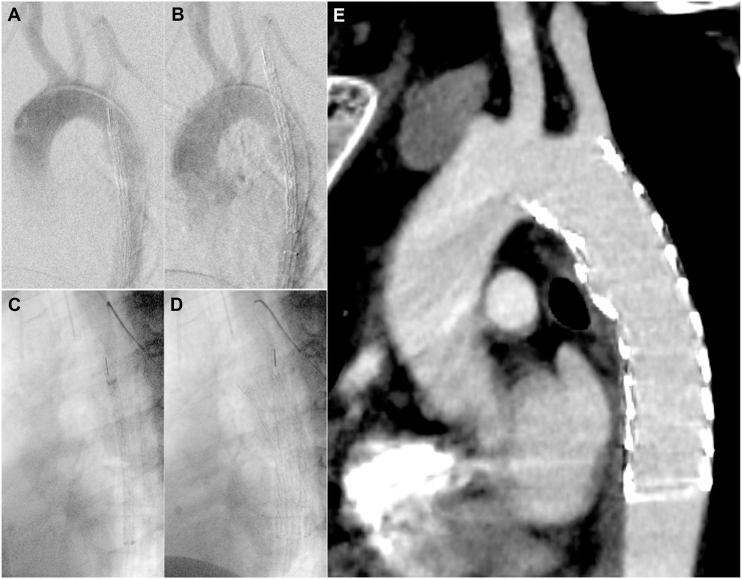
Fluoroscopic and postoperative computed tomography images. (A, B) After the proximal neck, the left subclavian artery (LSA) orifice and the site of aortic injury are confirmed using aortography, and the conformable GORE TAG (CTAG) (W. L. Gore & Associates) is carefully placed in the LSA. (C, D) The CTAG is positioned where its proximal gold marker is immediately distal to the LSA and then deployed. (E) The proximal landing length on the lesser-curvature side is adequate.
